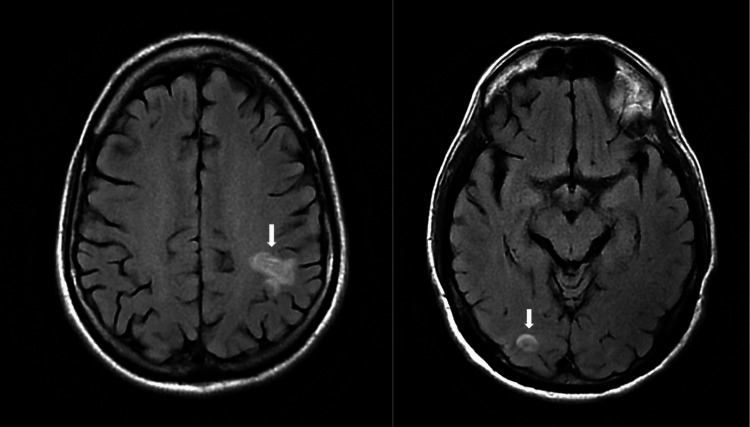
MRI brain axial FLAIR demonstrating septic emboli (arrows). MRI: magnetic resonance imaging; FLAIR: fluid-attenuated inversion recovery
